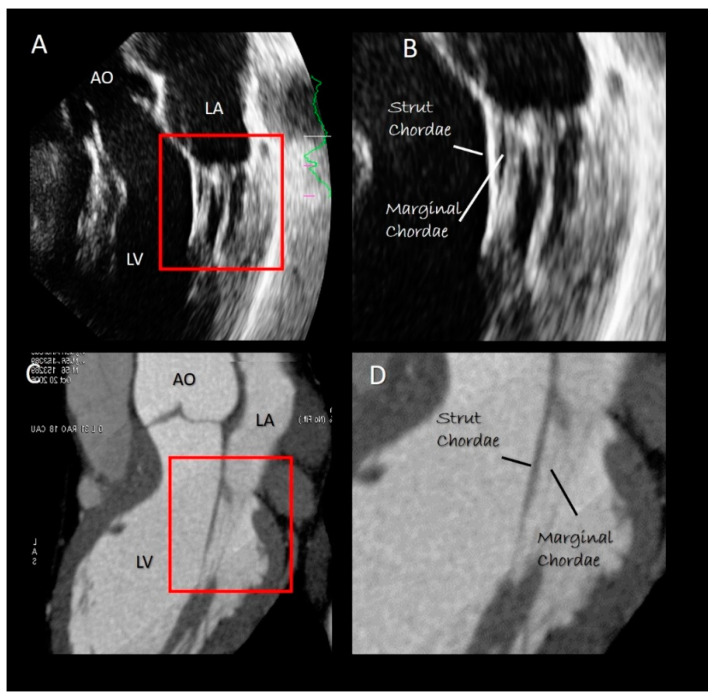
(A) 2D TTE and (C) CT cross section in long-axis view. (B,D) Magnified images of the structures inside the red rectangle of panel A and C, respectively, showing the strut and marginal chordae (see text).
